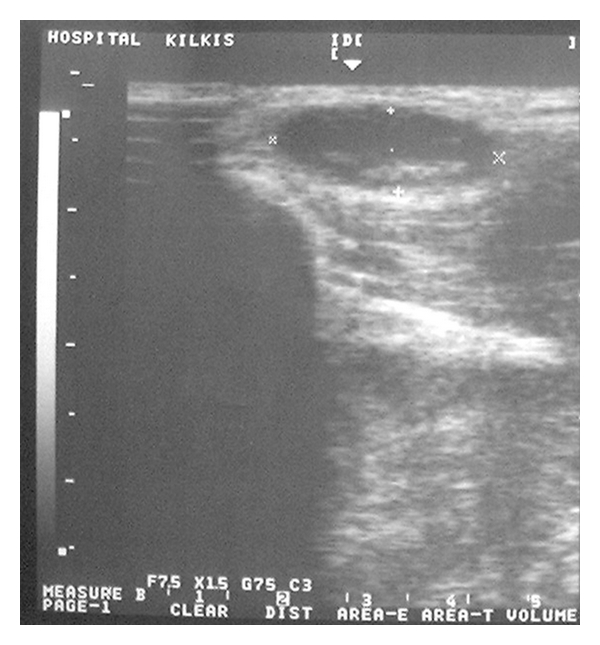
The (ECHO) ultrasonic scan of the area showing a sizeable infrasonic formation with distinct boundaries.
